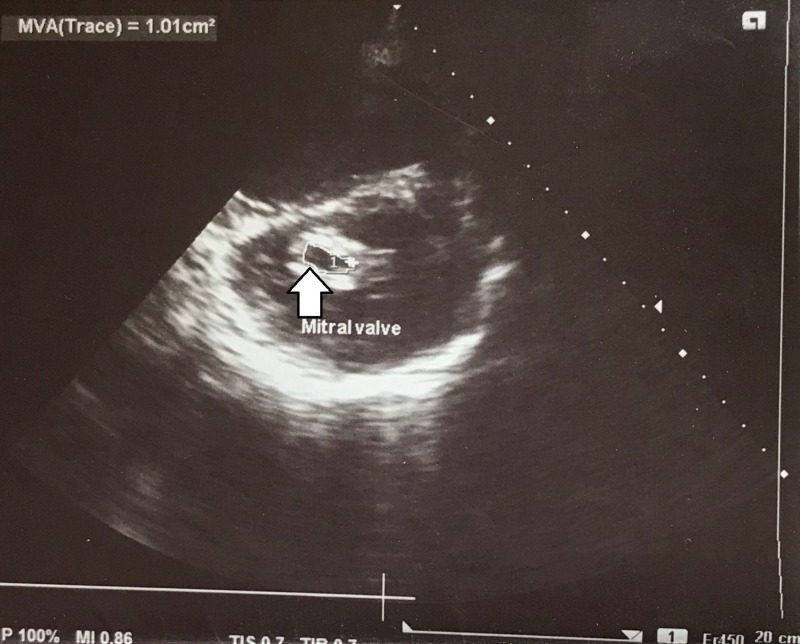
Transthoracic echocardiography on short axis view showing thickened, calcified mitral valve leaflets with MVA of 1.01 cm2 on planometry MVA: mitral valve area
